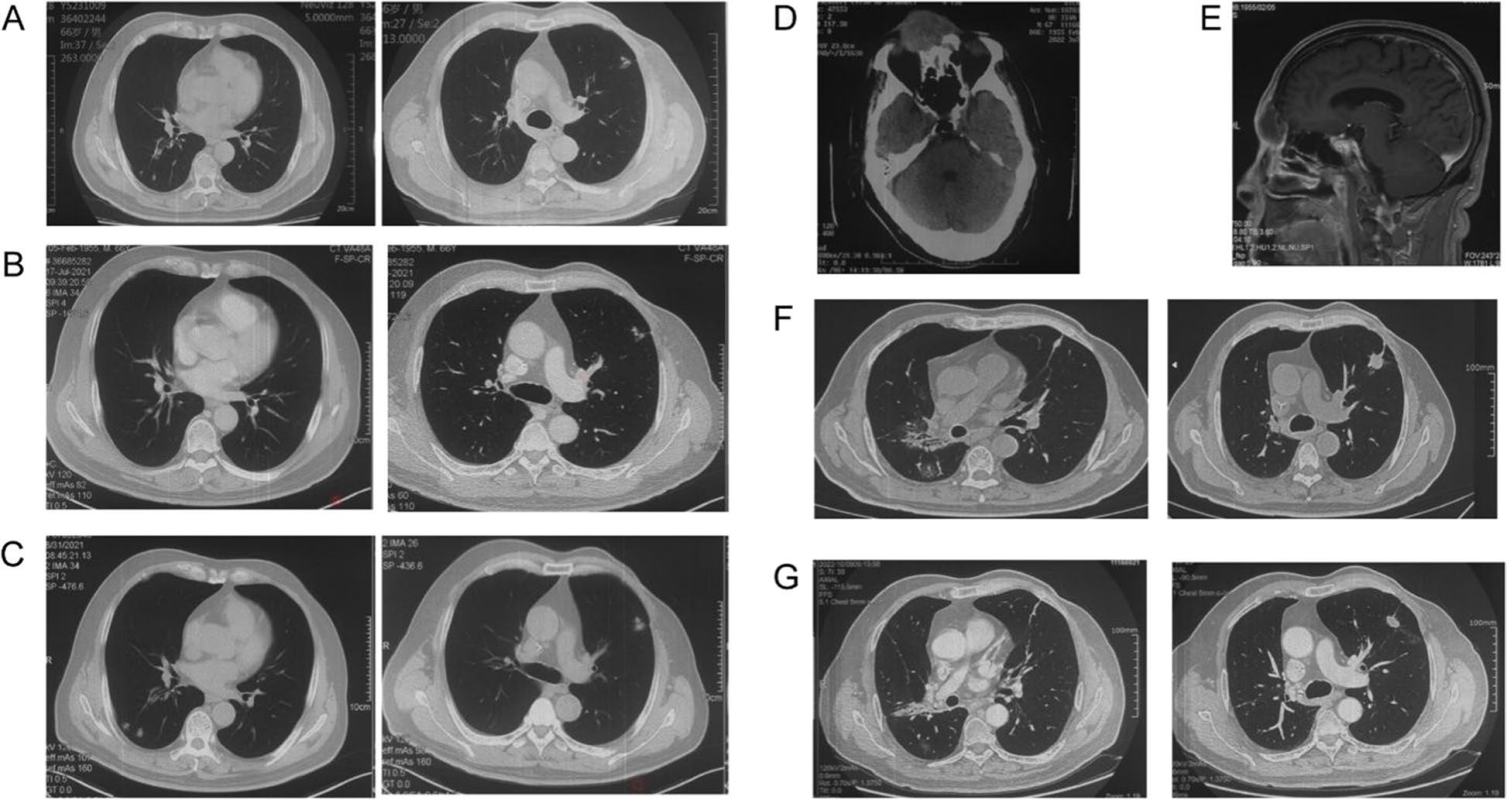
A Baseline CT image of lung metastases before olaparib treatment. B Chest CT image after 2 months olaparib treatment. C Chest CT image after 4 months olaparib treatment, the patient was evaluated as having stable disease. D Baseline MRI image of brain metastases before radiotherapy treatment. E MRI of brain metastases after 5 months radiation sequential fluzoparib therapy. F Chest CT after 3 months radiation therapy. G chest CT after 5 months radiation sequential fluzoparib therapy
